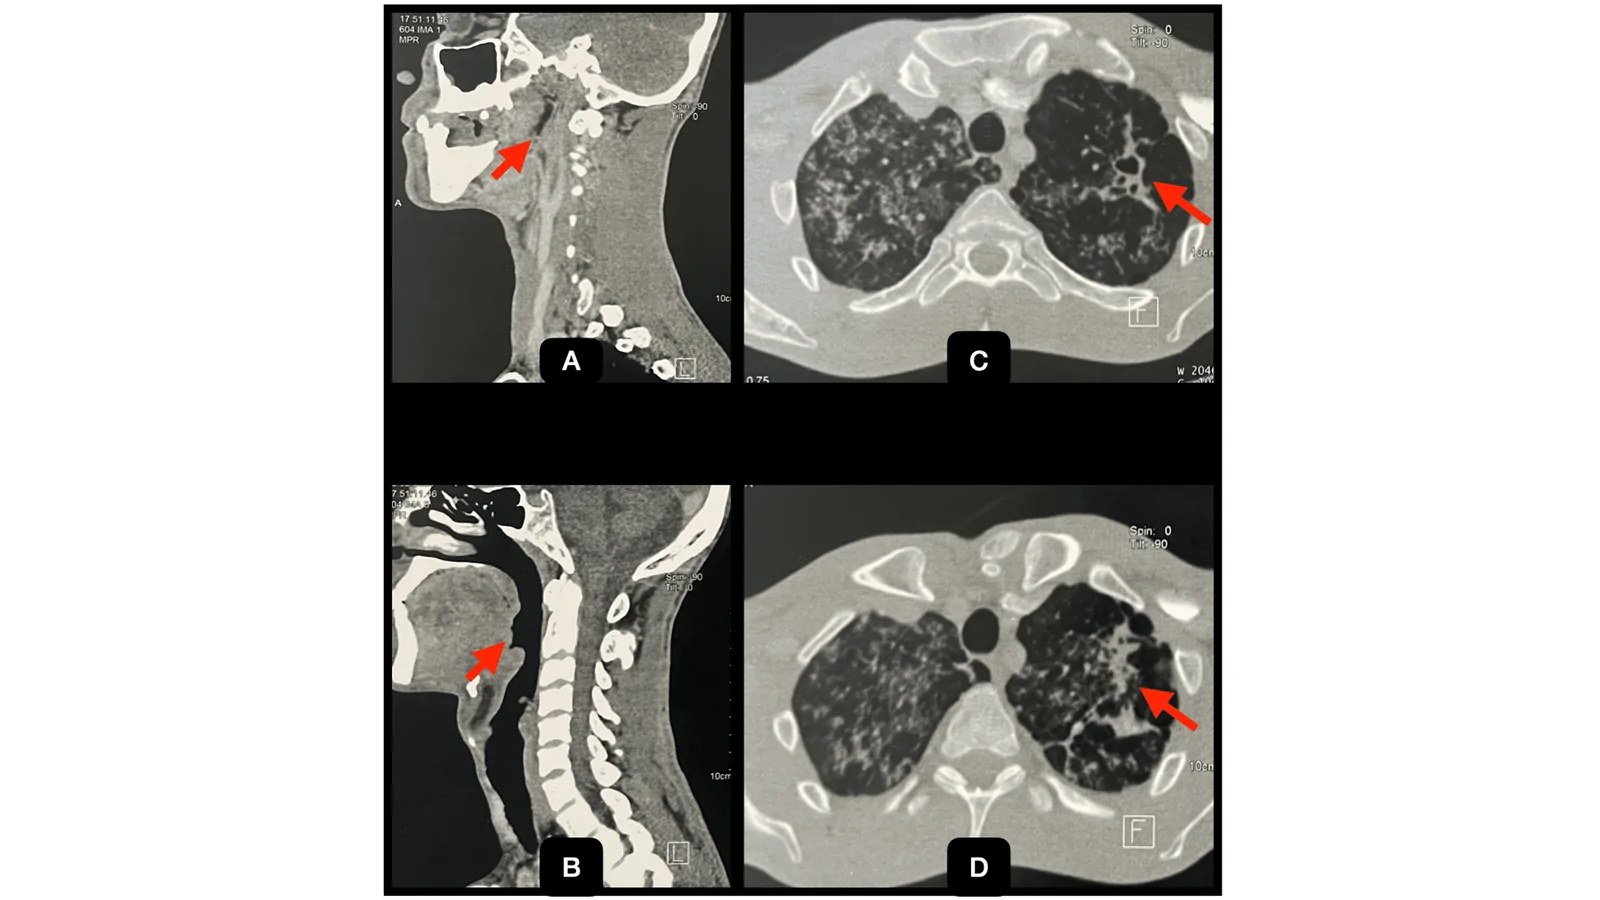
Contrast-enhanced cervicothoracic CT scan demonstrating pulmonary and tonsillar involvement (A-B) Oropharynx CT findings: tonsillar irregularity lining the palatine tonsils and epiglottic edema. (C-D) Pulmonary CT findings: bilateral apical pulmonary micronodules and a solid nodule associated with a cavitary lesion. CT: computed tomography
